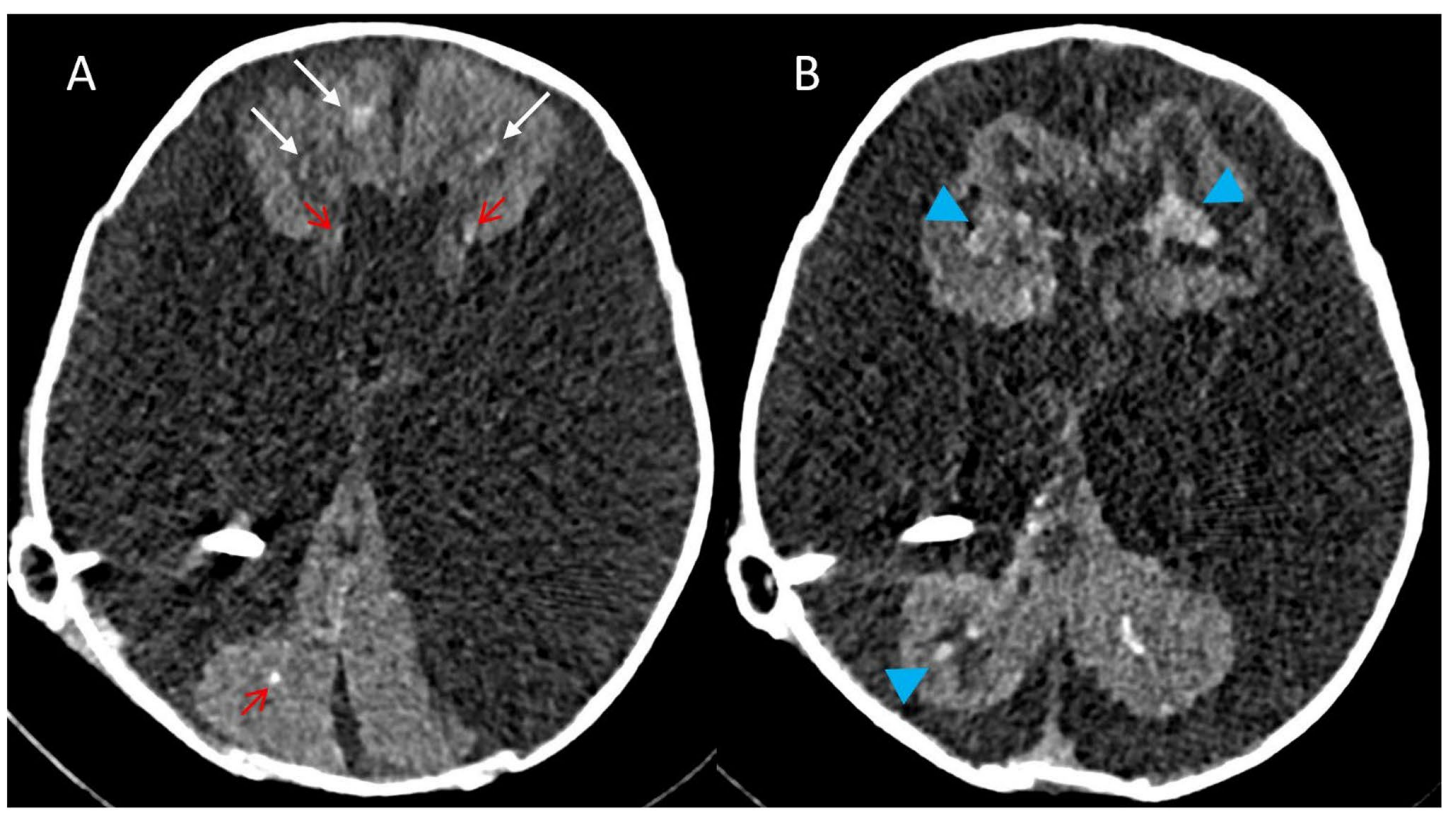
(a) Axial CT performed day 2 post right transparietal VP shunting for post-haemorrhagic hydrocephalus and porencephalic cysts due to an underlying COL4A1 mutation (same patient as Fig. 4) shows bilateral frontal WHIPS in A (white arrows) with evidence of ependymal and white matter calcifications (red arrows). (b) Axial CT performed 11 days later shows progression of bilateral frontal WHIPS and new right occipital WHIPS (blue arrowheads) surrounded by progressive low attenuation oedema. Bilateral enlarging CSF attenuation subdural collections are also evident in B distorting the parenchyma
